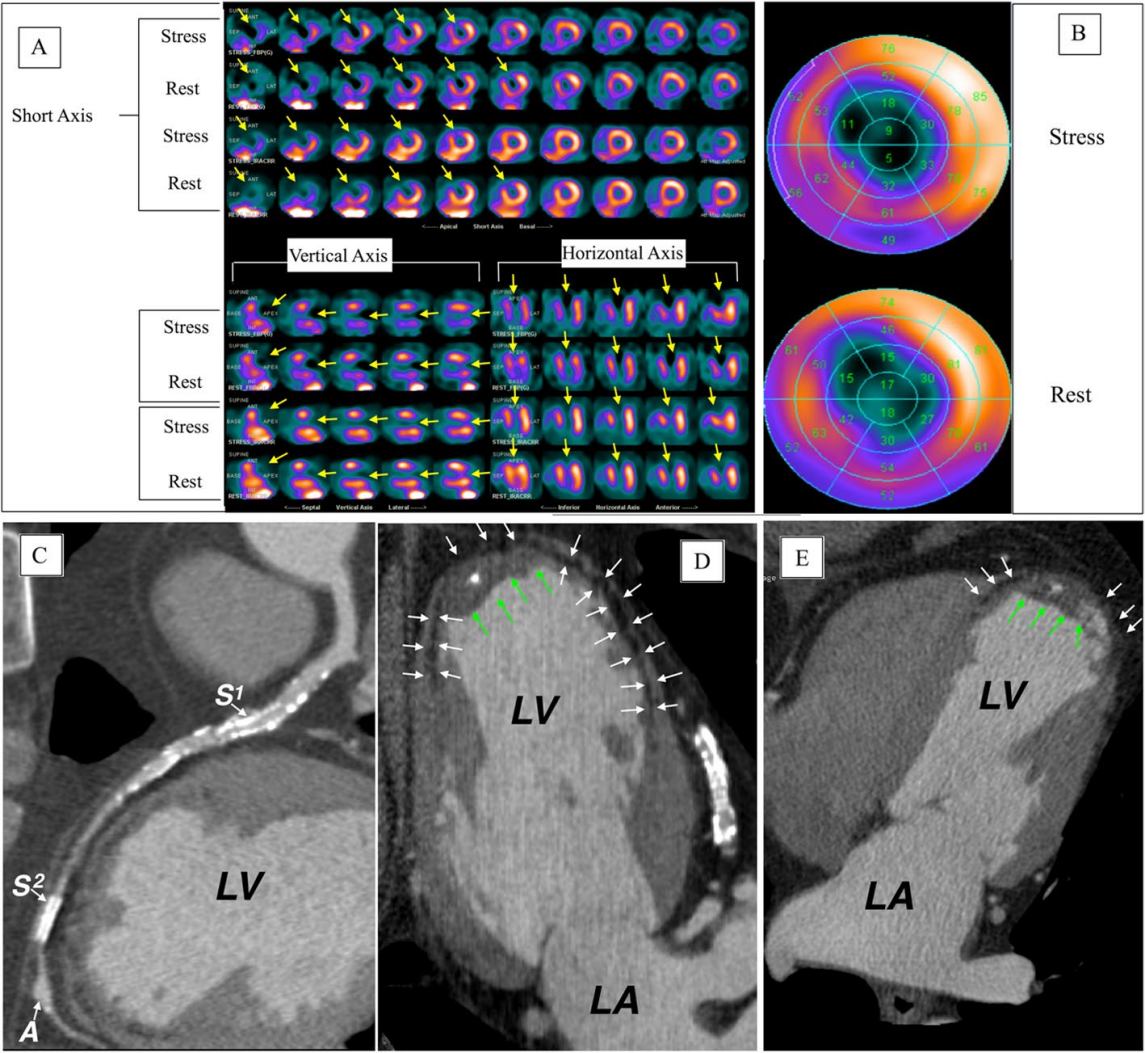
A SPECT images, B polar maps at stress (upper panel) and rest (lower panel), C–E computed tomographic angiography images. LV, left ventricle; LA, left atrium
